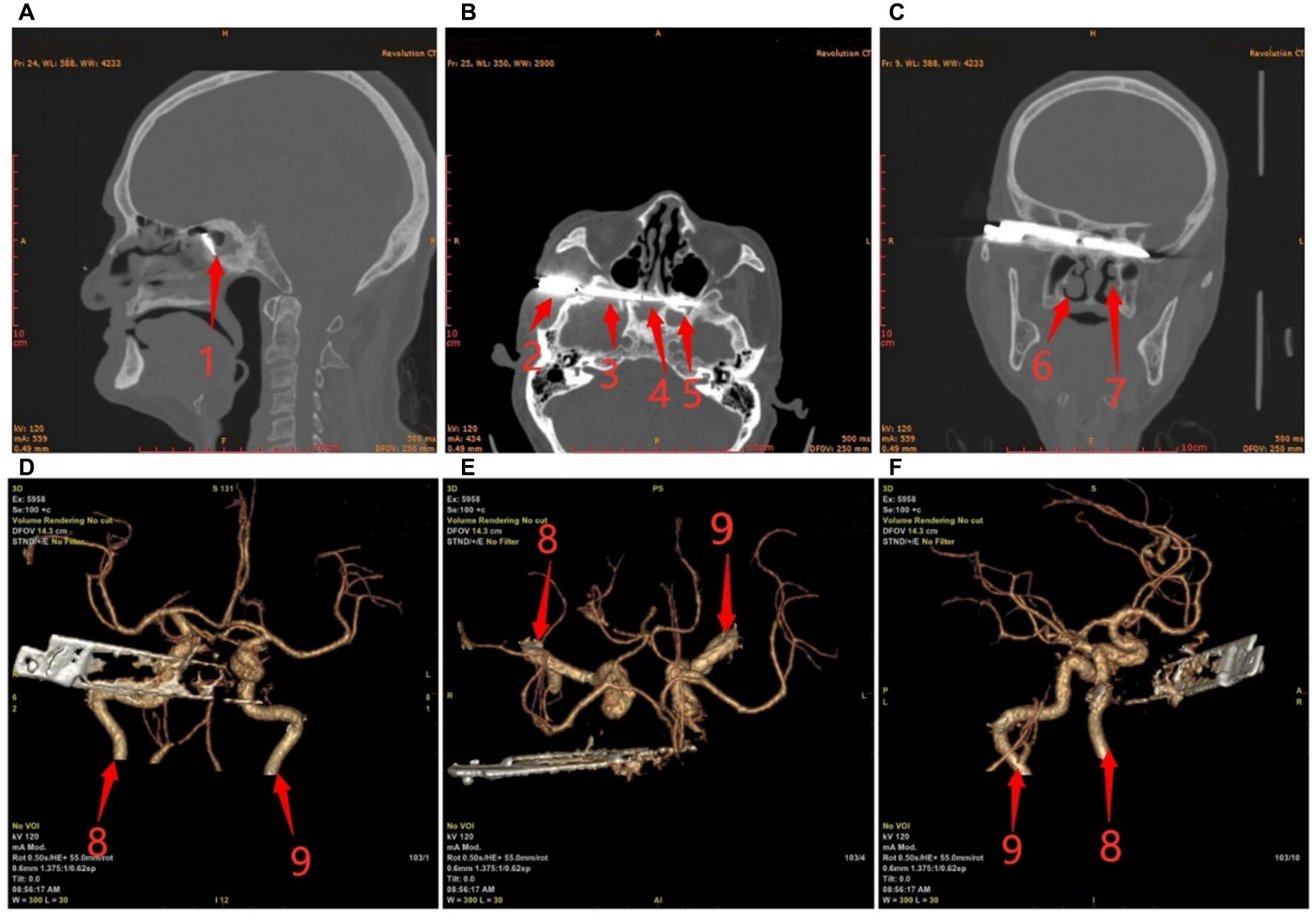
Preoperative craniocerebral CT and reconstruction. (A) Sagittal; (B) Transverse; (C) Coronal; (D–F) The positional relationship between blood vessels and knives. Red arrows: 1, sphenoid sinus; 2, temporal fossae; 3, the base of the middle cranial fossa; 4, sphenoid sinuses (right); 5, sphenoid sinus (left); 6, right nasal cavity; 7, left nasal cavity; 8, right internal carotid artery; and 9, left internal carotid artery.
